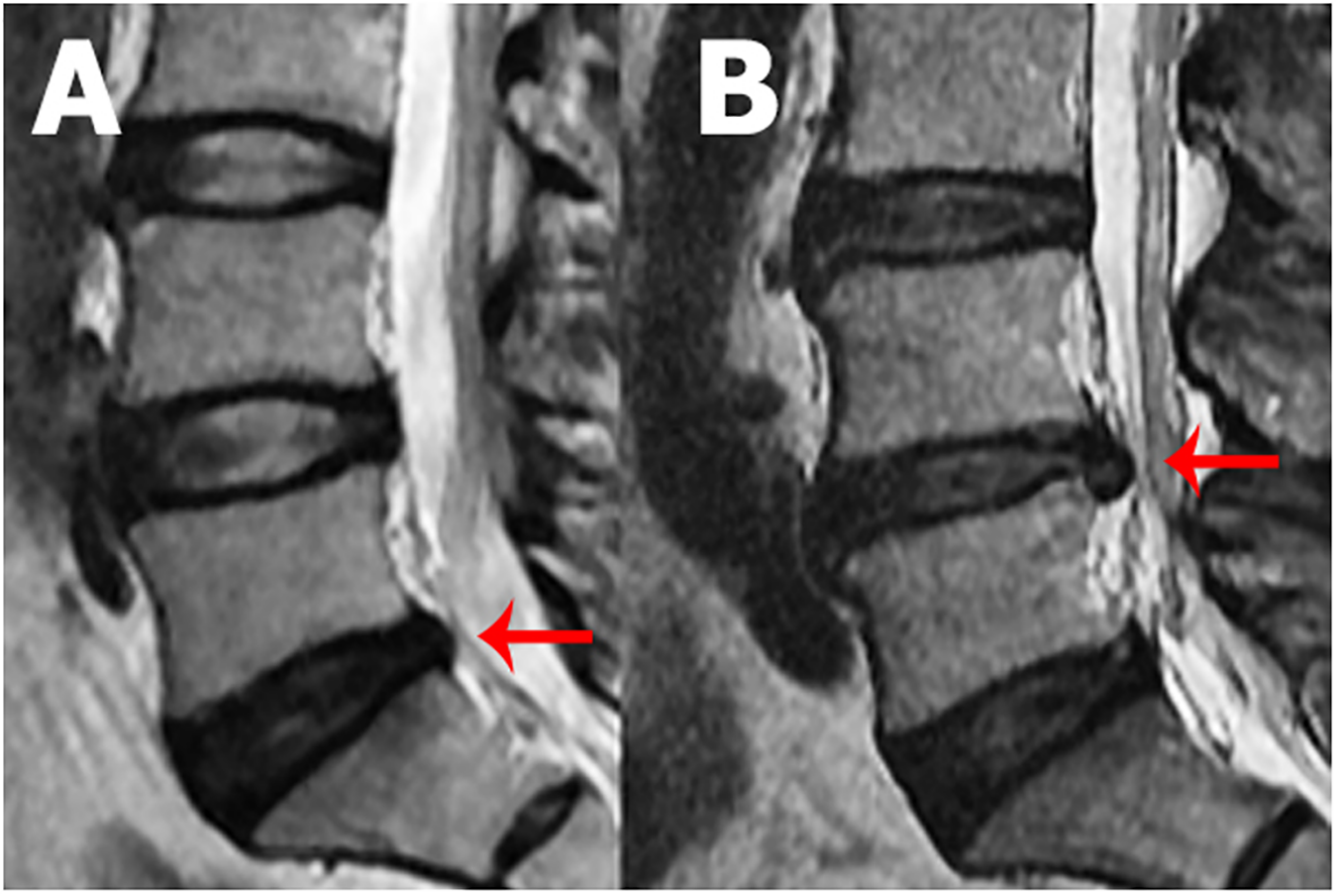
Sagittal MRI of the lumbar spine showing disc protrusion at L5/S1 (A) and disc extrusion at L4/L5 (B).
